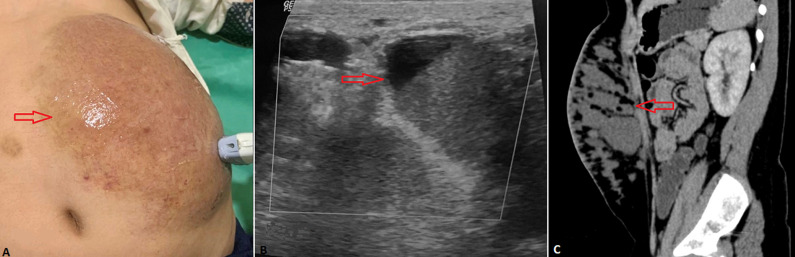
A) large mild reddish-blue smooth lesion occupying the left side of the abdomen (red arrow); B) ultrasound image of the lesion showing multiple cystic lesions with internal debris within (red arrow); C) CT scan sagittal section shows multiple soft tissue density lesion communicating with each other in the subcutaneous plane of abdomen (red arrow); findings consistent with abdominal lymphatic malformation- lymphangioma
